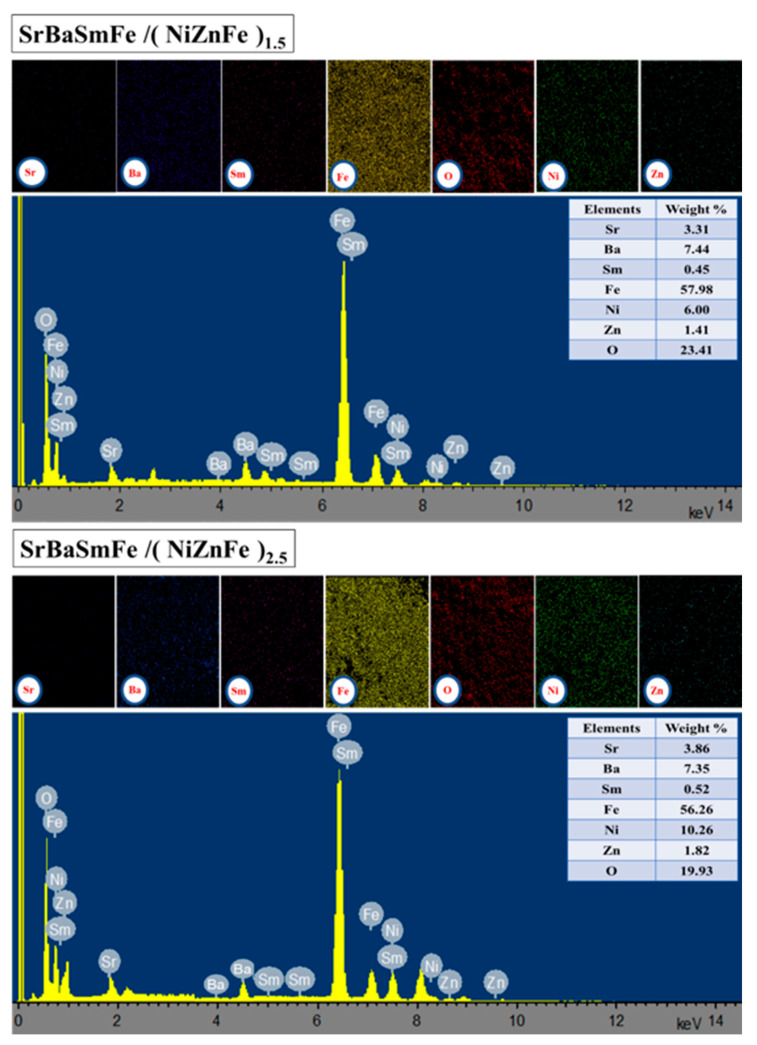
Energy-dispersive X-ray (EDX) and elemental mapping of hard/soft ferrite SrBaSmFe/(NiZnFe)_x_ NCs with *x* = 1.5 and 2.5.
